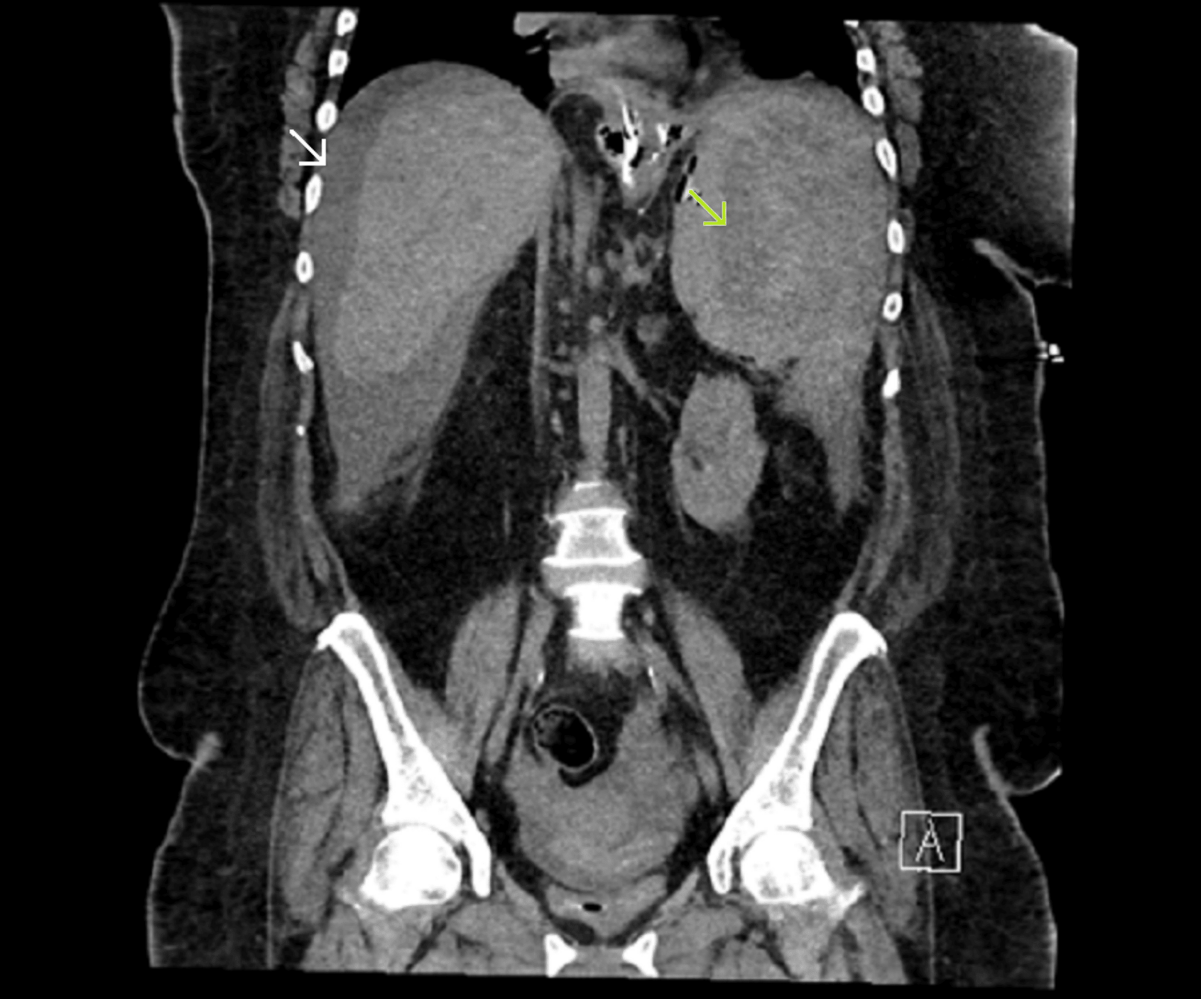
Repeat CT scan, day one post-diagnostic laparoscopy Green arrow indicating ruptured splenic capsule. White arrow indicating increasing intra-abdominal free fluid. CT, computed tomography
